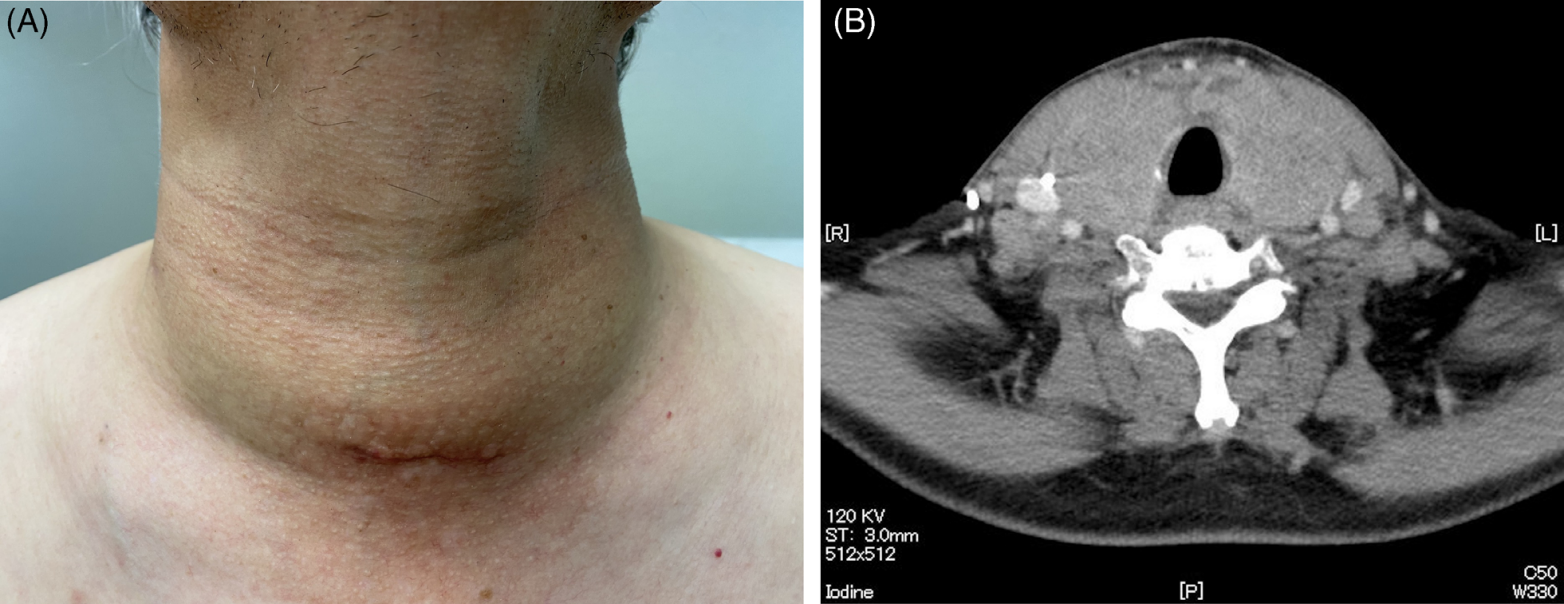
(A) Photography of the neck shows diffuse goitre enlargement. (B) Contrast‐enhanced computed tomography of the neck and chest revealed diffuse goitre enlargement
